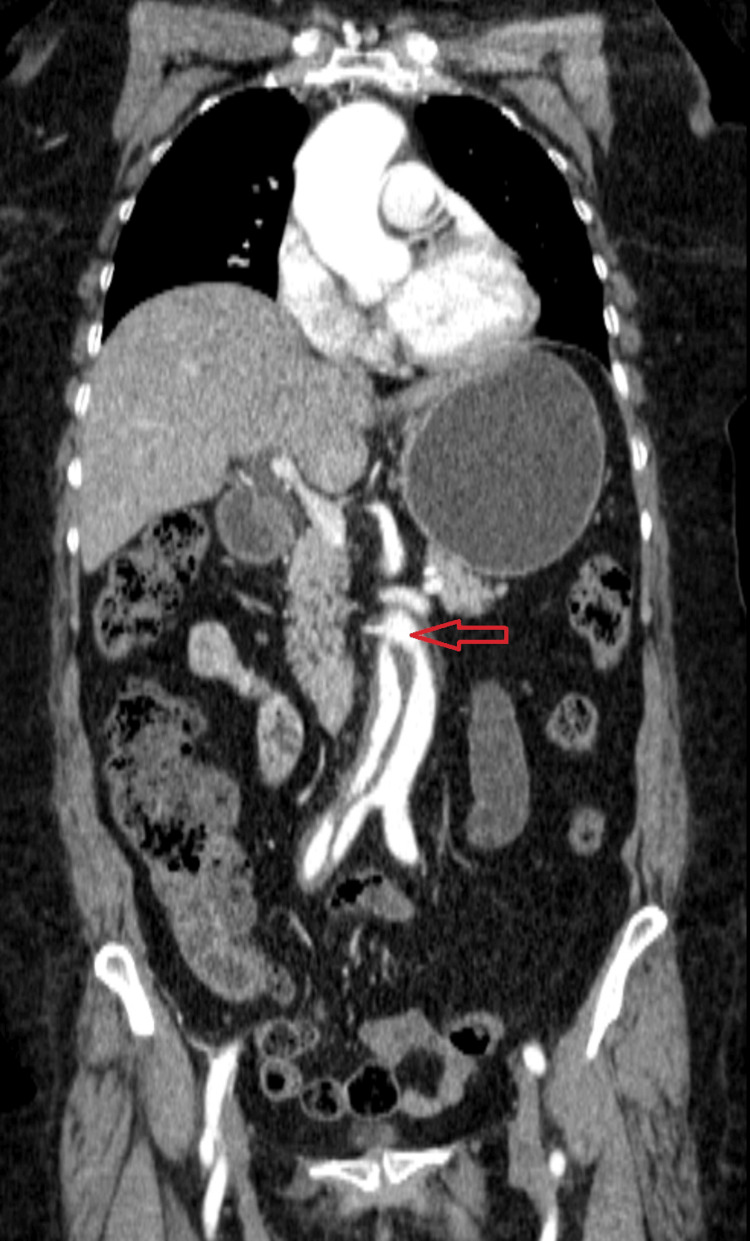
Coronal contrast-enhanced CTA demonstrating an infrarenal abdominal aortic dissection The image shows an intimal flap (red arrow) within the infrarenal aorta, just distal to the renal arteries, resulting in a double-lumen appearance characteristic of aortic dissection and confirming the infrarenal origin of the lesion. CTA, CT angiography
